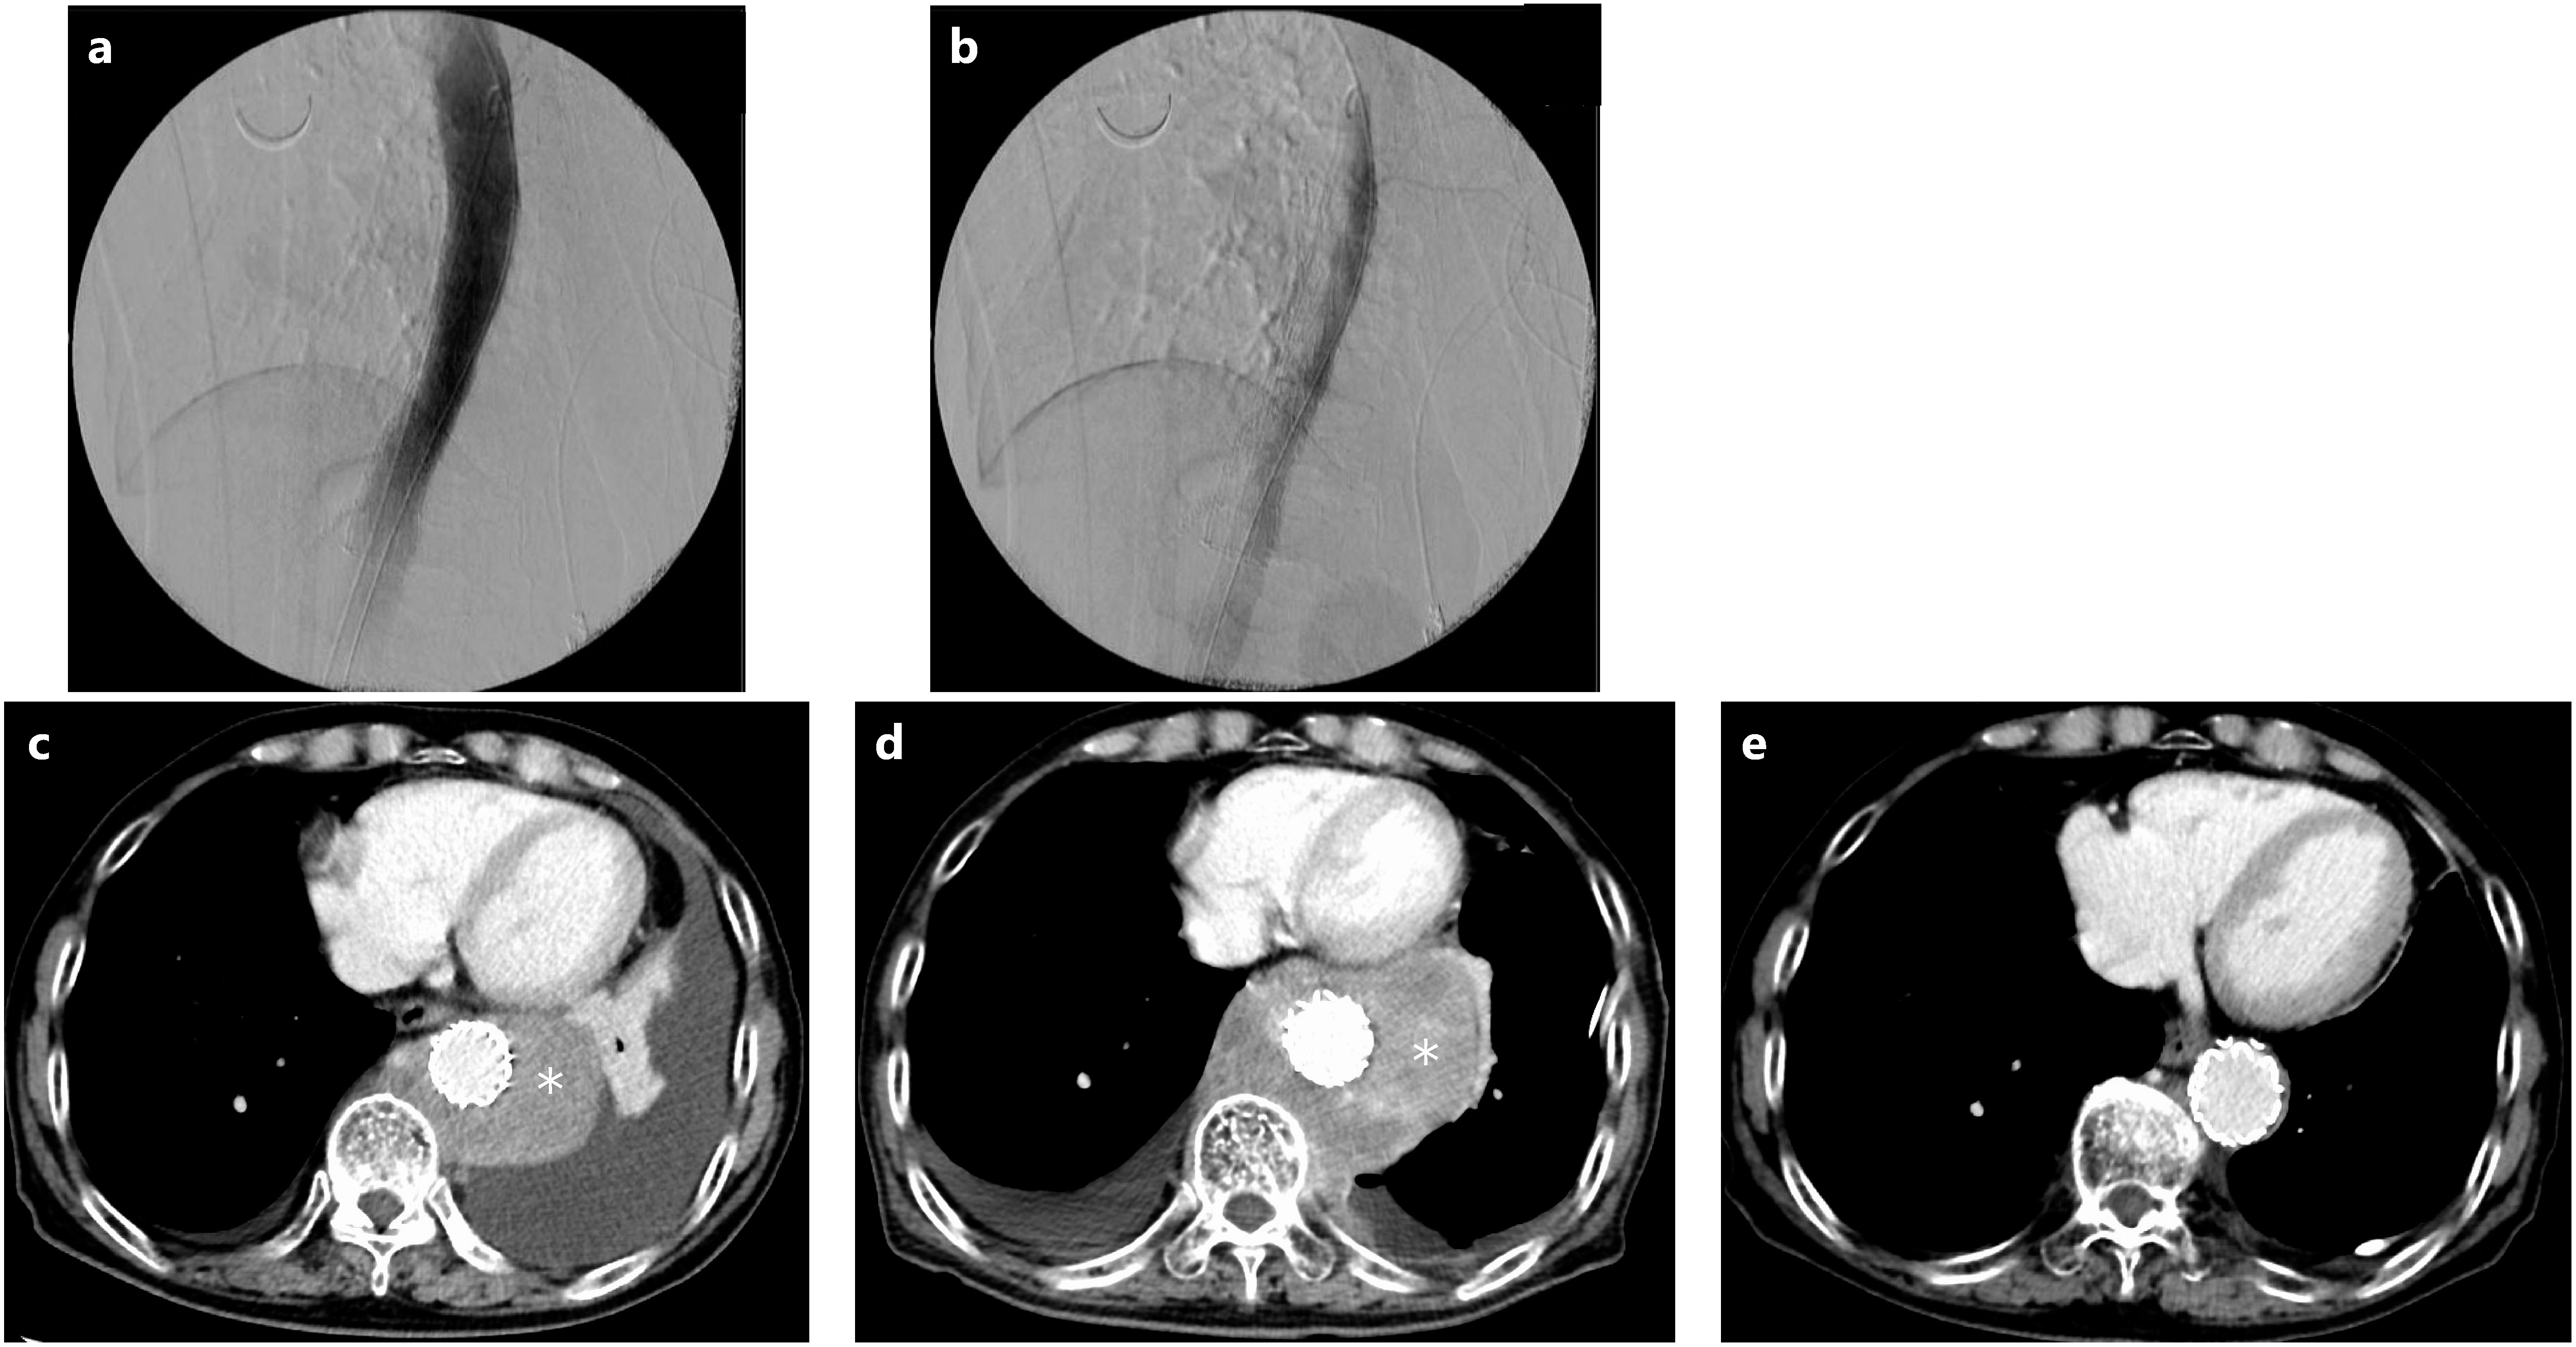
Fig. 2 Intraoperative angiography and computed tomography (CT) images. a and b. Images of the completion angiography; no endoleak nor feeding artery-like finding to the neoplasm at the early phase (a) and late phase (b). c. Postoperative CT showed progression of the thoracic aneurysm with increased left pleural effusion (arrowhead) without an endoleak. d. Re-enhanced CT showed that the aortic aneurysm around the stent graft enlarged irregularly with heterogenous contrast enhancement. e. The aneurysm-like structure around the stent graft disappeared without any findings of a fistula or rupture on the contrast-enhanced-CT after chemotherapy. The asterisk indicates a false lumen-like structure.
